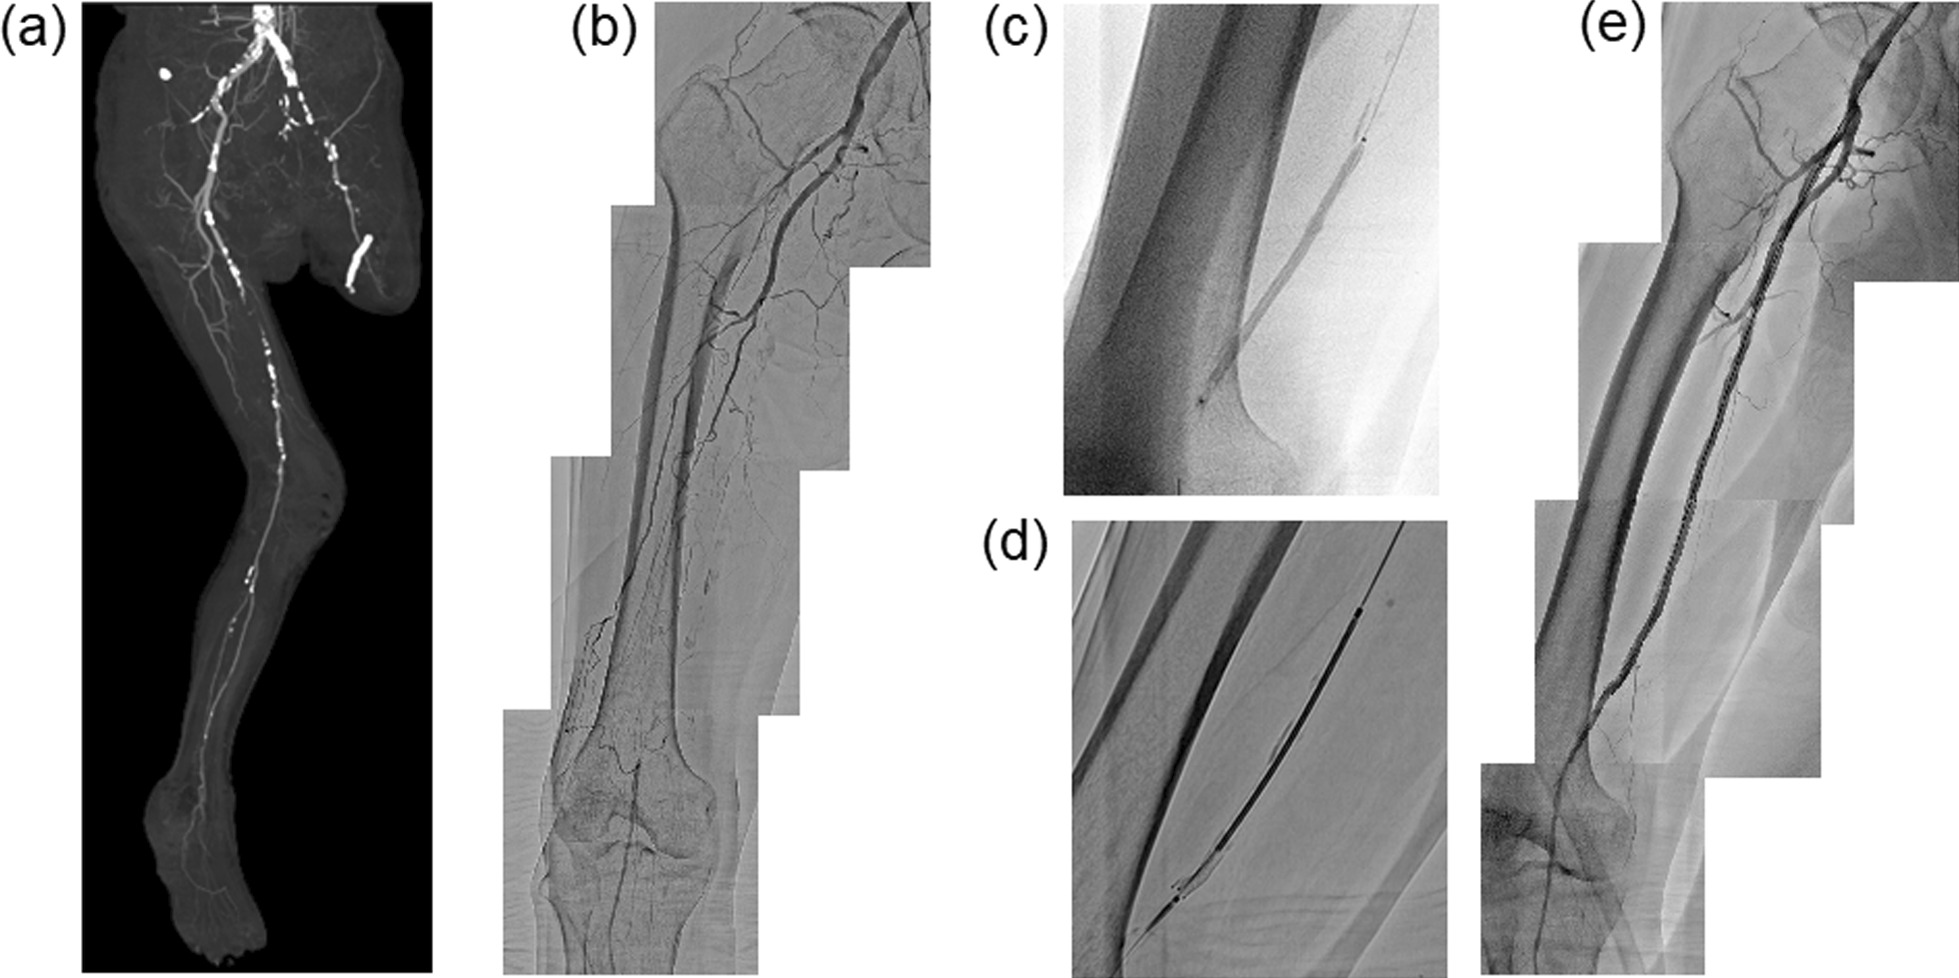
Computed tomography (CT) angiographic image showing total occlusion of the left superficial femoral artery (SFA) and the left iliac artery (a). Angiography of the right SFA before and after peripheral vascular intervention (PVI), shown in b–e. Initial angiography showing total occlusion of the left SFA from the ostium to the distal portion (b). PVI was performed with balloon inflation c and stent implantation (d). Final angiography after PVI showing a good result (e)
